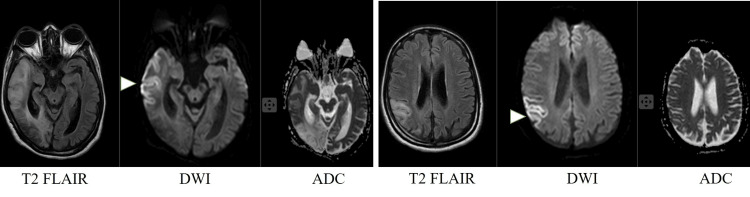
MRI scan of the brain revealing GRD Arrowheads reveal areas of GRD as hyperintensities on DWI with corresponding hypointensities in ADC images. GRD: Gyriform restricted diffusion, DWI: Diffusion-weighted imaging, ADC: Apparent diffusion coefficient
